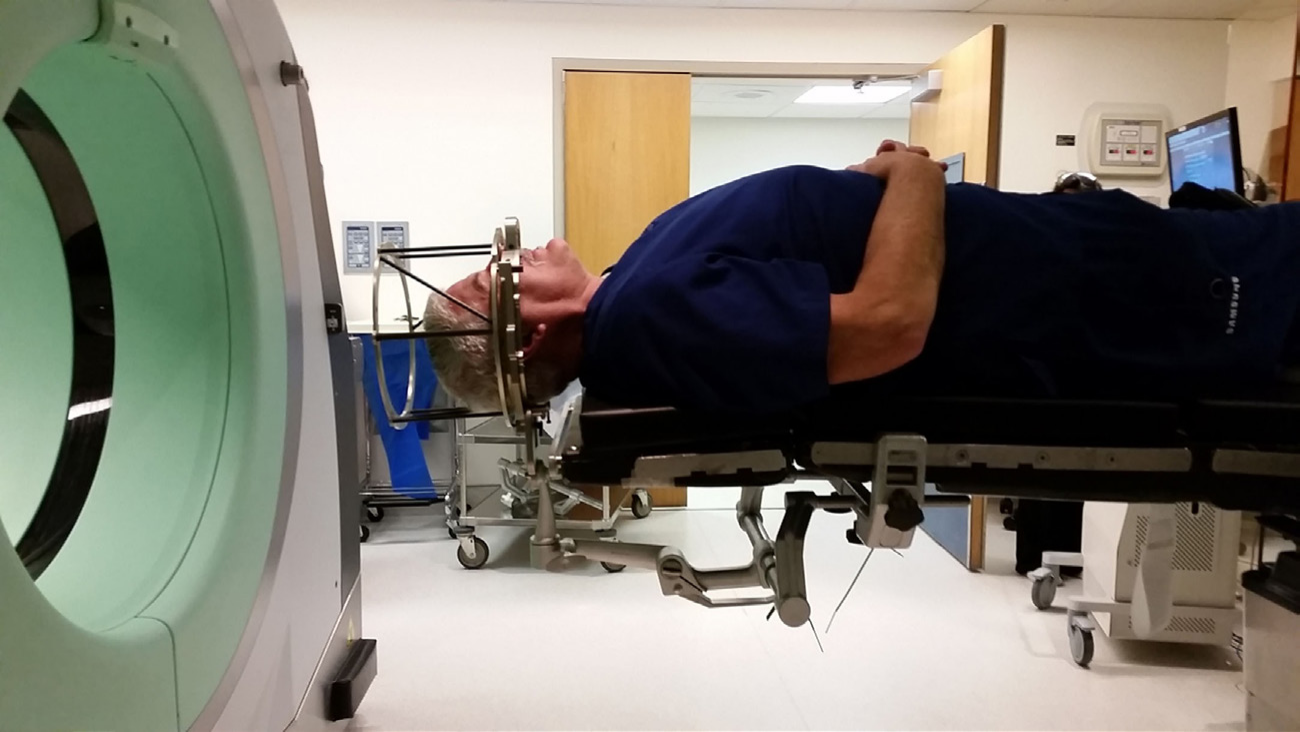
Positioning of potential patient in CRW frame, prior to CT scanning.
